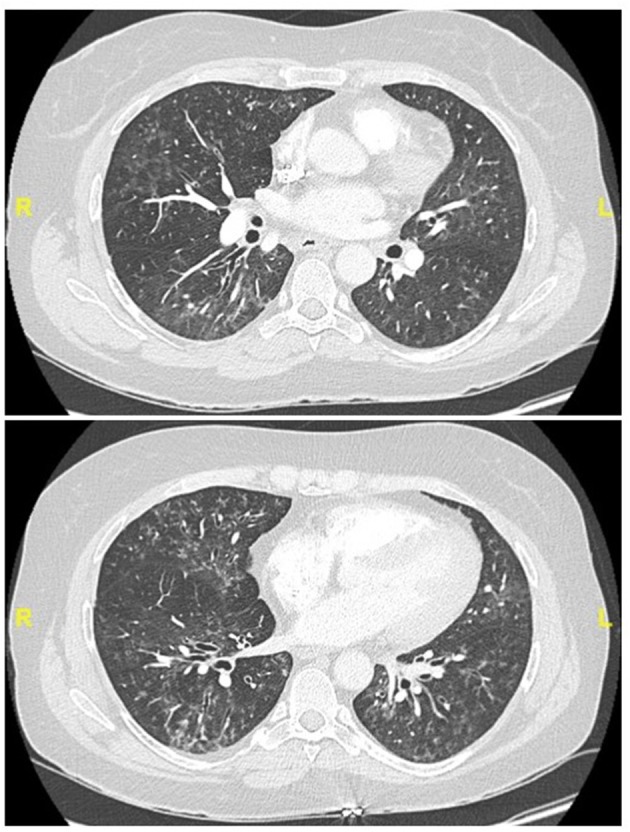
Chest CT (lung window) in a 55-year old, non-smoker female patient fulfilling IPAF criteria, with Raynaud' phenomenon (clinical domain) and a complex pattern of non-specific interstitial pneumonia and lymphocytic bronchiolitis at biopsy (multicompartment involvement, morphologic domain).
